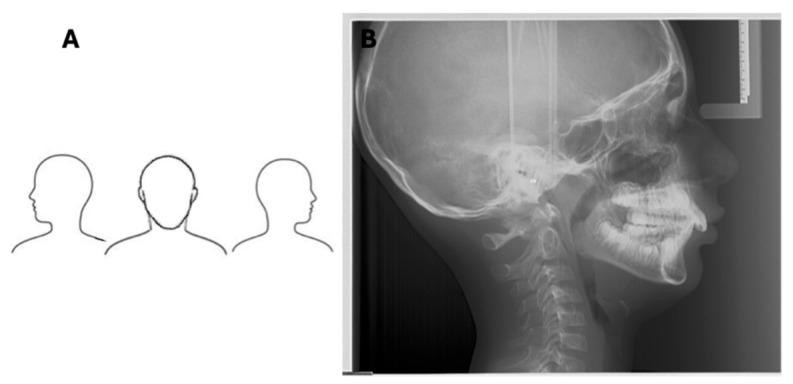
Rolling. (A) Picture. (B) LCR. Acquired with direct digital device. Head roll results in the asymmetric projection of craniofacial structures of the right and left sides. These structures do not appear overlapping on the radiograph but are misaligned in the antero-posterior direction. Such misalignment is evident at the molar teeth and mandibular bone profiles. Acquisition parameters: 100 kVp, 20 mA, scanning time ~4 s.
